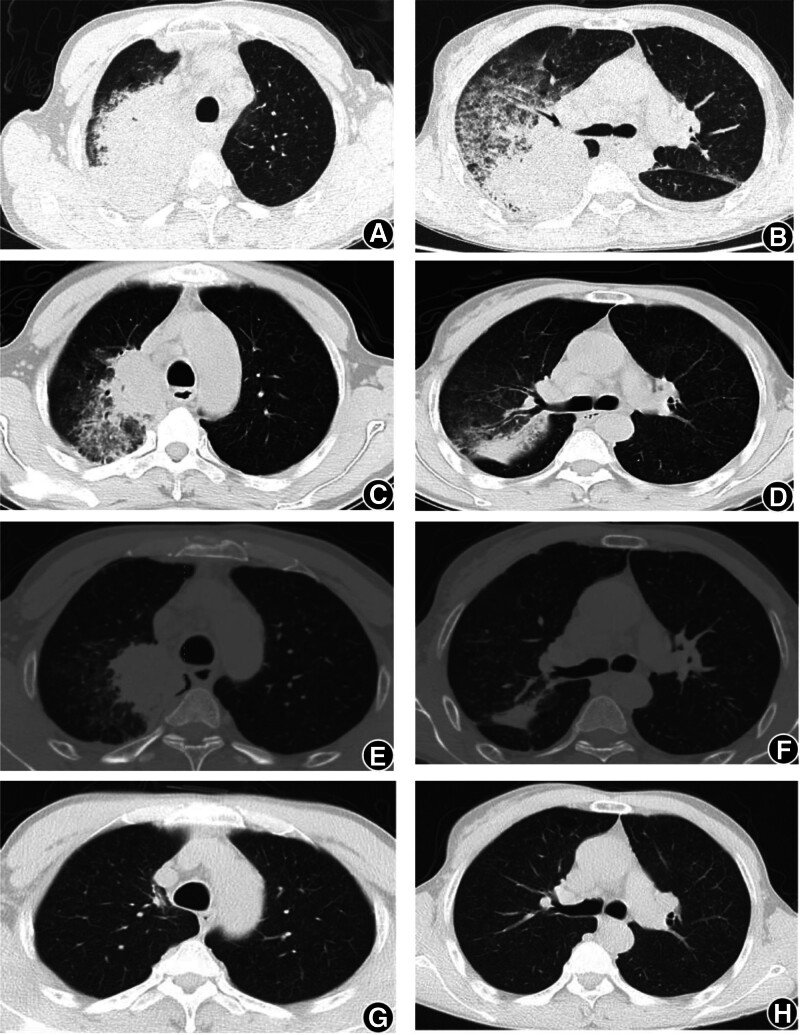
The chest CT scan findings for the patient: (A, B) dated September 30, 2020; (C, D) dated October 12, 2020; (E, F) dated October 22, 2020; and (G, H) dated July 19, 2021.
